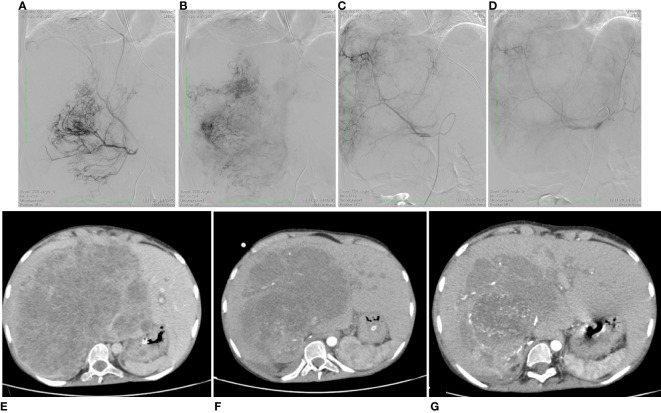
Angiographic imaging of the UESL-supplying vessels and MRI images showing favorable dynamics of the liver tumor volume. (A) Proximal portion of the right diaphragmatic artery supplying UESL; (B) distal portion of the right diaphragmatic artery supplying UESL; (C) hepatic artery proper before chemoembolization; (D) hepatic artery proper after chemoembolization, with the catheter left in place for chemoperfusion. (E) before the interventional procedures; (F) after the first session of chemoembolization; (G) after the second session of chemoembolization.
